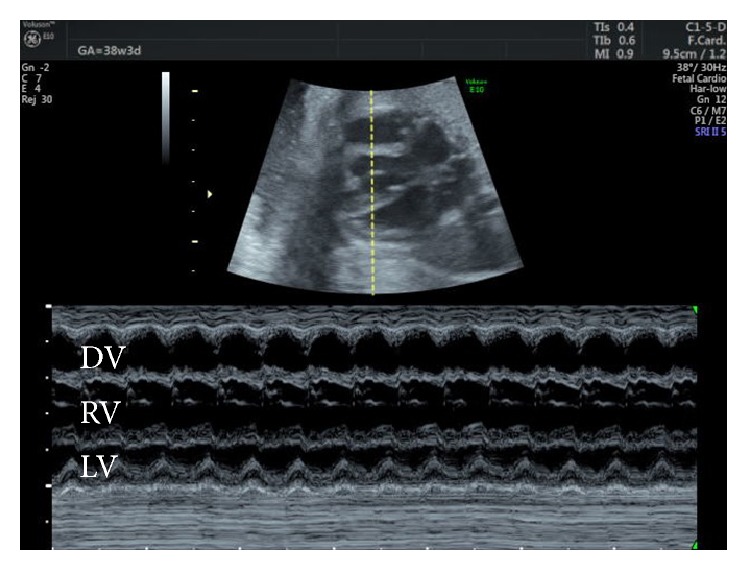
Fetal M-mode ultrasound: four-chamber view showing the diverticulum, right ventricle, and left ventricle. DV, diverticulum; LV, left ventricle; RV, right ventricle.
